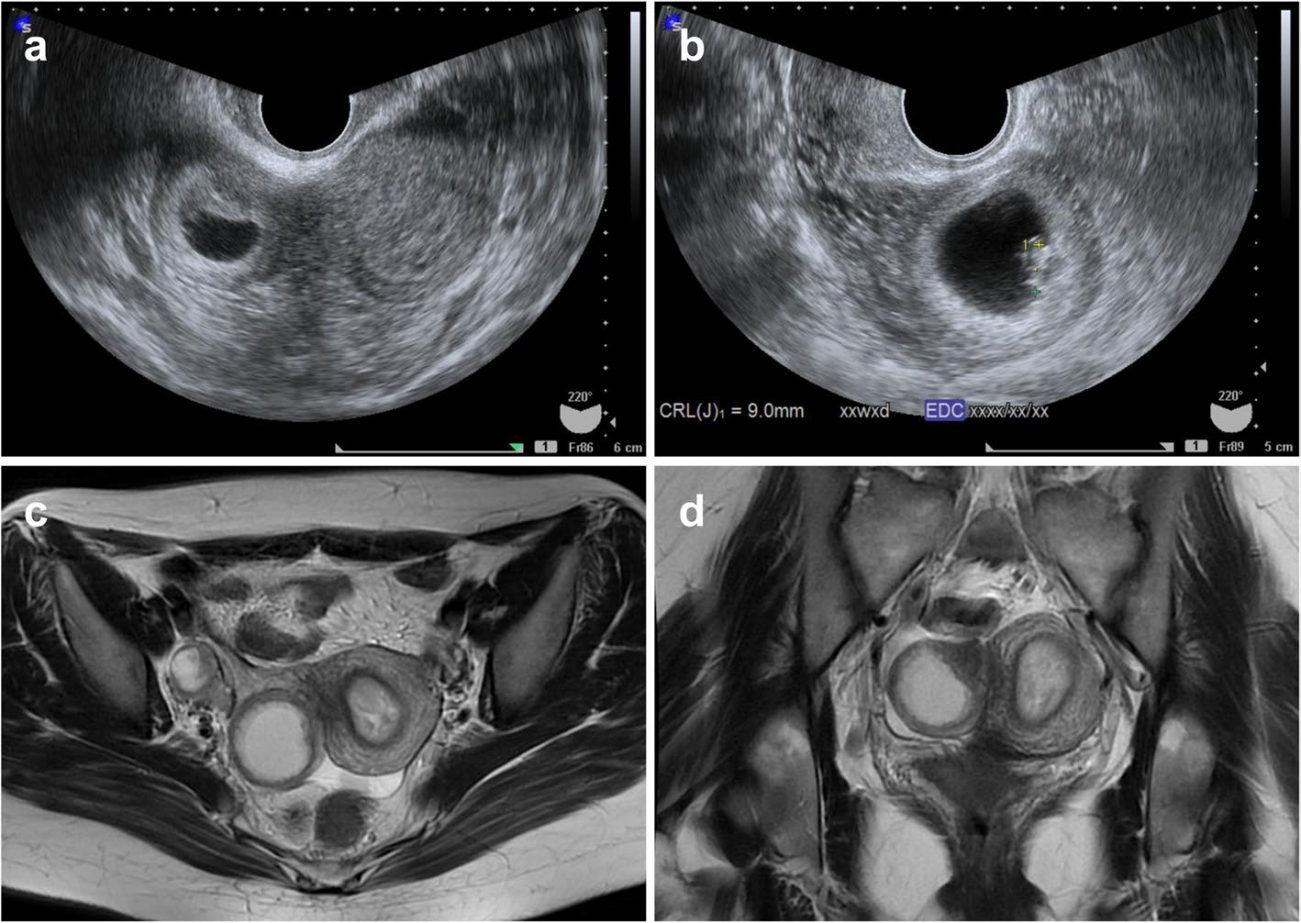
Images showing a left unicornuate uterus with a right noncommunicating rudimentary horn pregnancy. On transvaginal ultrasonography, a a gestational sac was observed in the right rudimentary horn, and thickened endometrium was observed in the left unicornuate uterus at 6 weeks of gestation; b a fetus of 9.0 mm size with a heartbeat was observed at 7 weeks of gestation. c T2-weighted transverse magnetic resonance imaging at 7 weeks of gestation d T2-weighted coronal magnetic resonance imaging at 7 weeks of gestation
